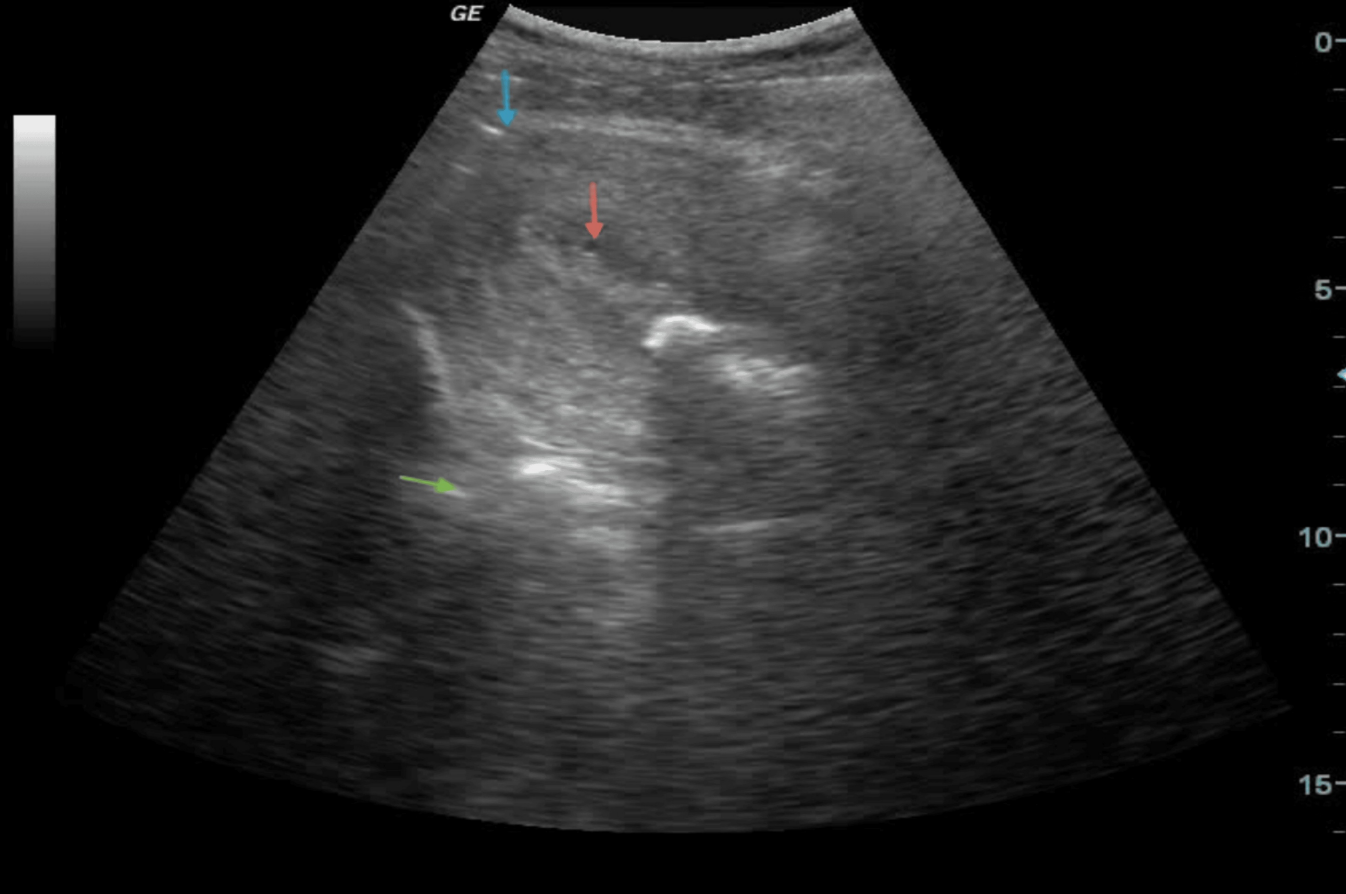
Abdominal ultrasound examination 18 hours post-delivery. blue arrow: uterine fundus; red arrow: chitosan gauze; green arrow: acoustic shadow
